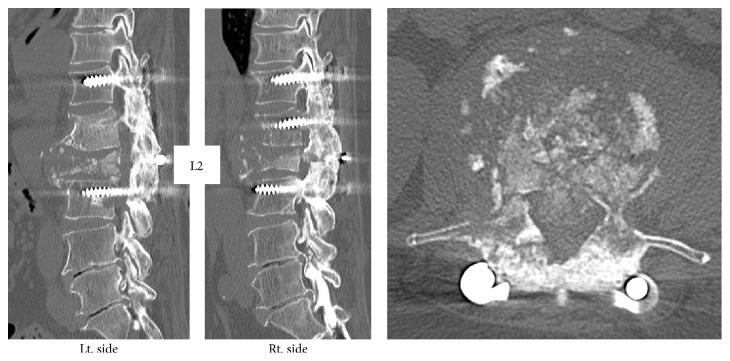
CT images. Vertebral arch (T12–L3) after posterior fixation surgery indicated complete bone union and bridge formation.
